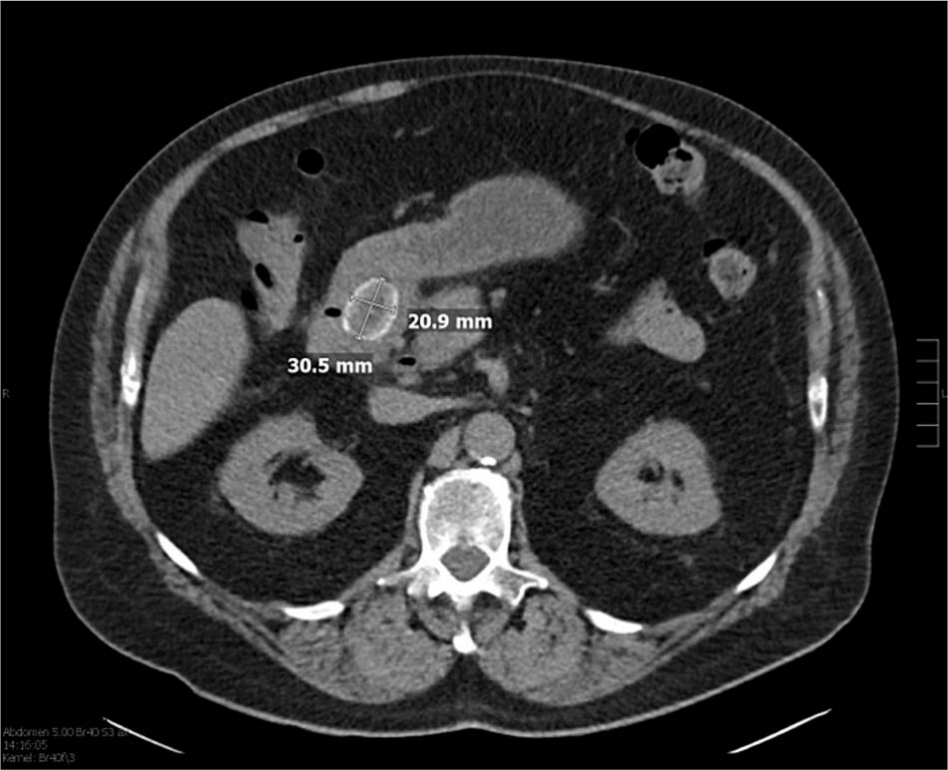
CT of the abdomen without contrast showing biliary gas, suspicious for cholangitis, in addition to the previously mentioned stone.
